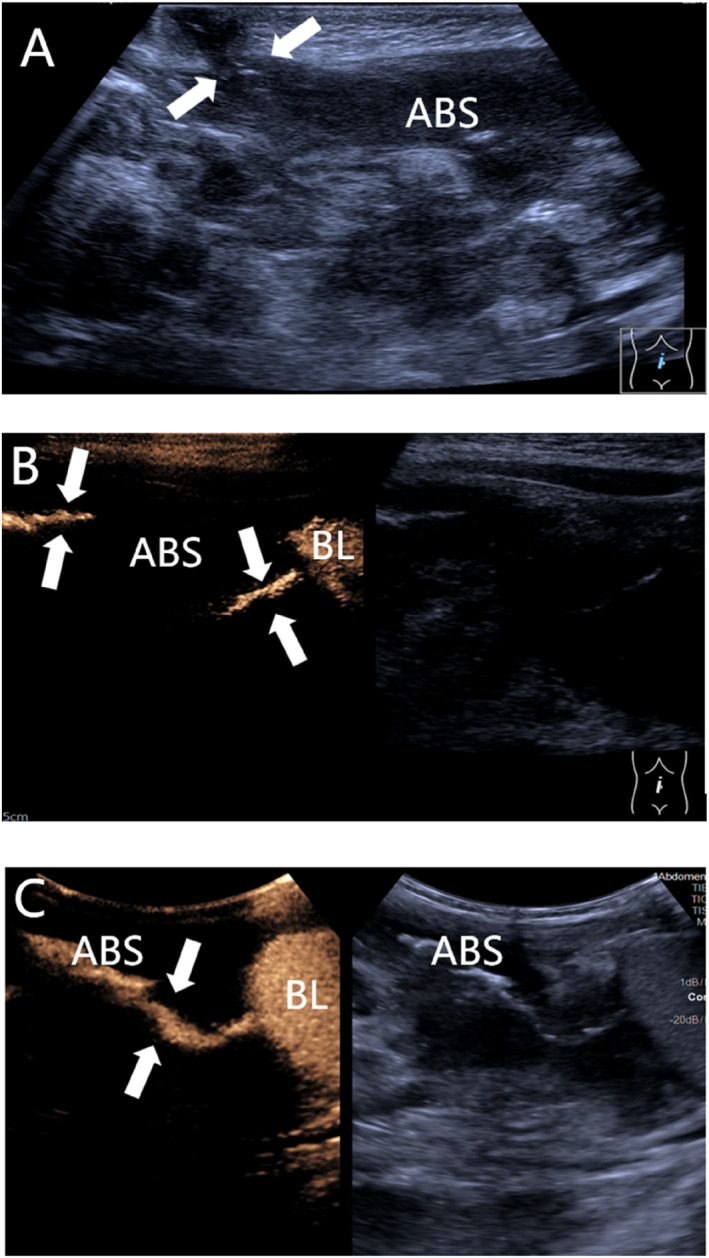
A 1.5‐year‐old male, clinically diagnosed with an umbilical intestinal fistula combined with a vesicoileal fistula. (A) Conventional ultrasound image showing an abnormal channel in the umbilicus (arrowheads) connecting to an intra‐abdominal abscess (ABS). (B) Real‐time transumbilical contrast‐enhanced ultrasound (CEUS) showing that the contrast agent reached the abdominal abscess cavity (ABS) through the fistula (arrowheads), and at the same time, the bladder (BL) connected to the abdominal abscess cavity (ABS) via a fistula (arrowheads). (C) CEUS via the bladder showing that the contrast agent reached the abdominal abscess cavity (ABS) via a fistula (arrow).
